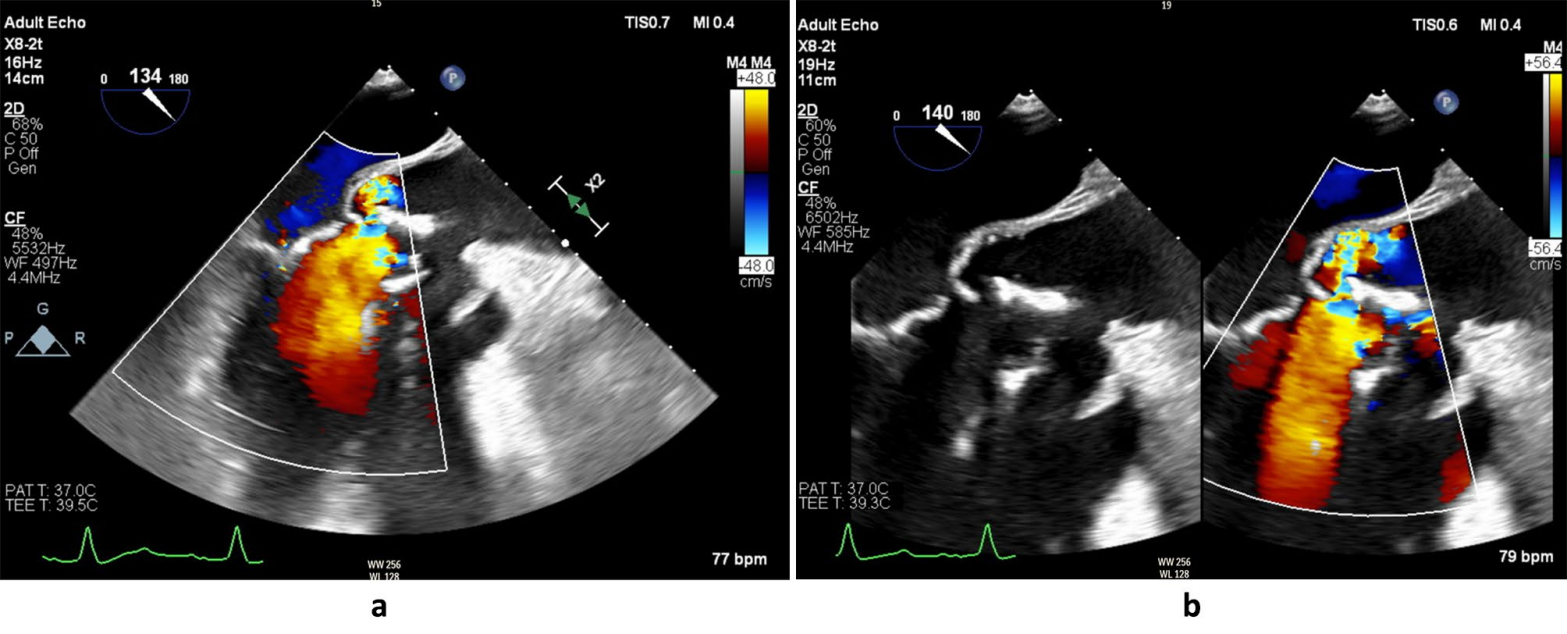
a & b: Transoesophageal echocardiogram long axis view of the dilated sinus of valsalva, aortic regurgitation and rocking of the aortic valve
